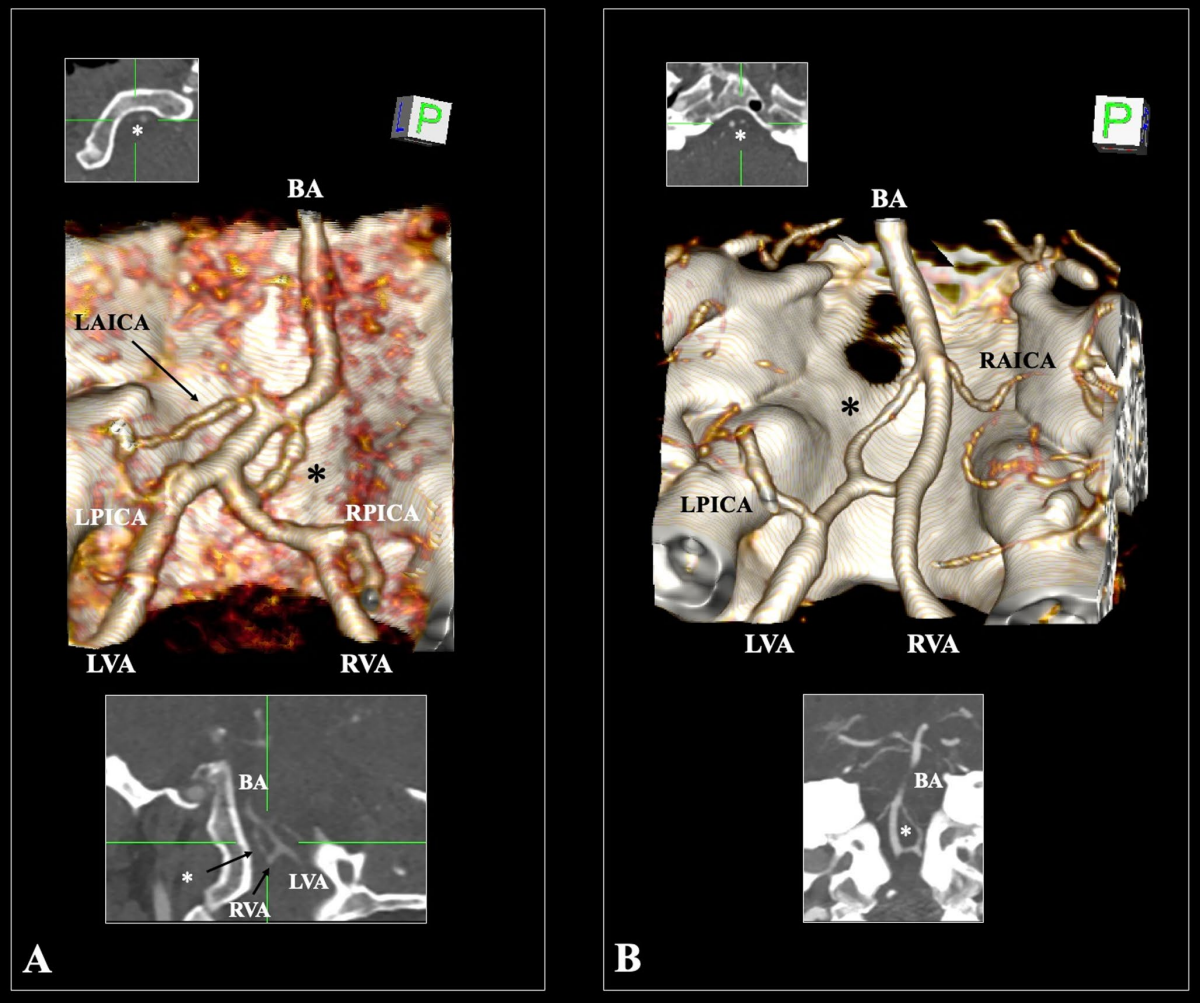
A Three-dimensional volume-rendered and multiplanar reconstructed (MPR) computed tomography angiography (CTA) images demonstrating a rare arterial ring at the vertebrobasilar junction (VBJ) in Case #1. The left and right vertebral arteries (LVA and RVA) join to form the basilar artery (BA). A variant arterial branch (marked with asterisks- *) arises from the RVA and reconnects with the BA approximately 3.4 mm distal to its origin, forming a complete arterial ring. The posterior inferior cerebellar arteries (PICA) originate normally from the V4 segments of both VAs. The left anterior inferior cerebellar artery (LAICA) is also visible coursing laterally. B CTA images of Case #2 showing an arterial ring configuration at the VBJ. The LVA and RVA merge to form the BA. A variant arterial branch (marked with an asterisk- *) originates from the LVA and reanastomoses with the BA distal to its origin, forming a distinct vascular ring. The left PICA (LPICA) and right anterior inferior cerebellar artery (RAICA) originate from their typical locations. MPR reconstructions confirm the complete continuity of the ring
